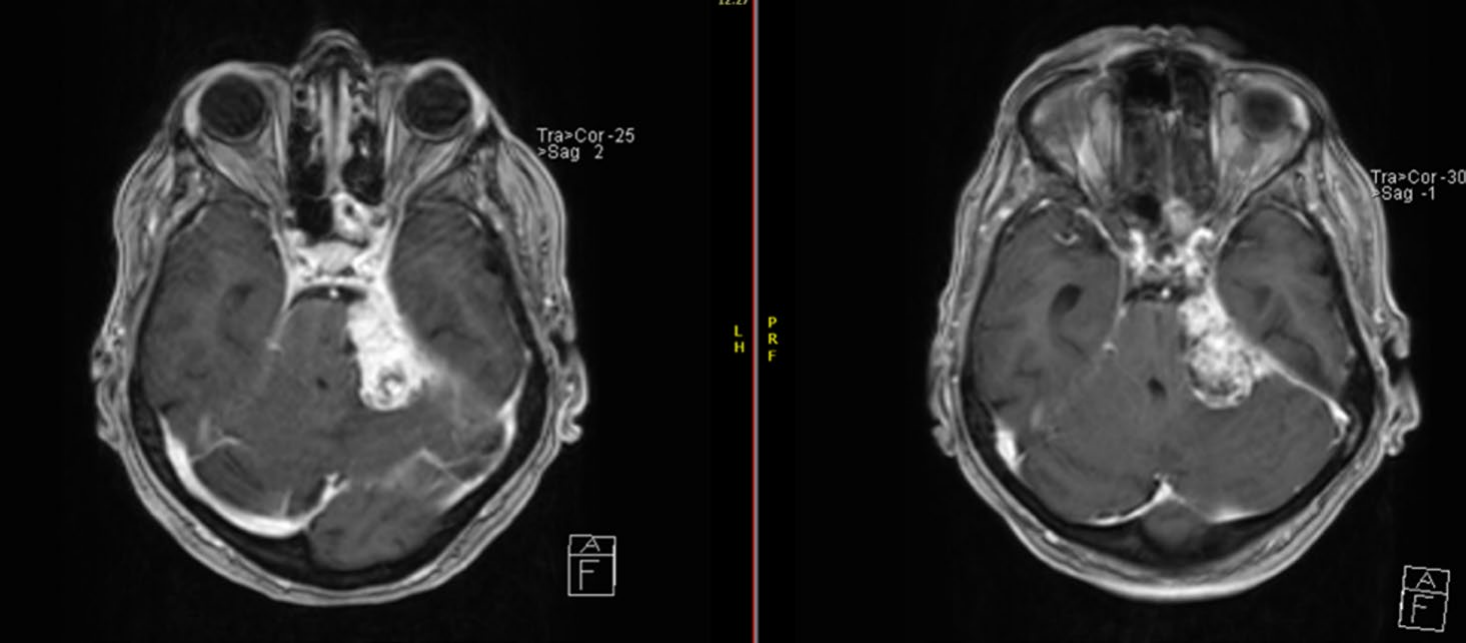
Axial T1-weighted contrast-enhanced MRI scans of a patient with a skull base meningioma before (left) and after (right) radiotherapy
